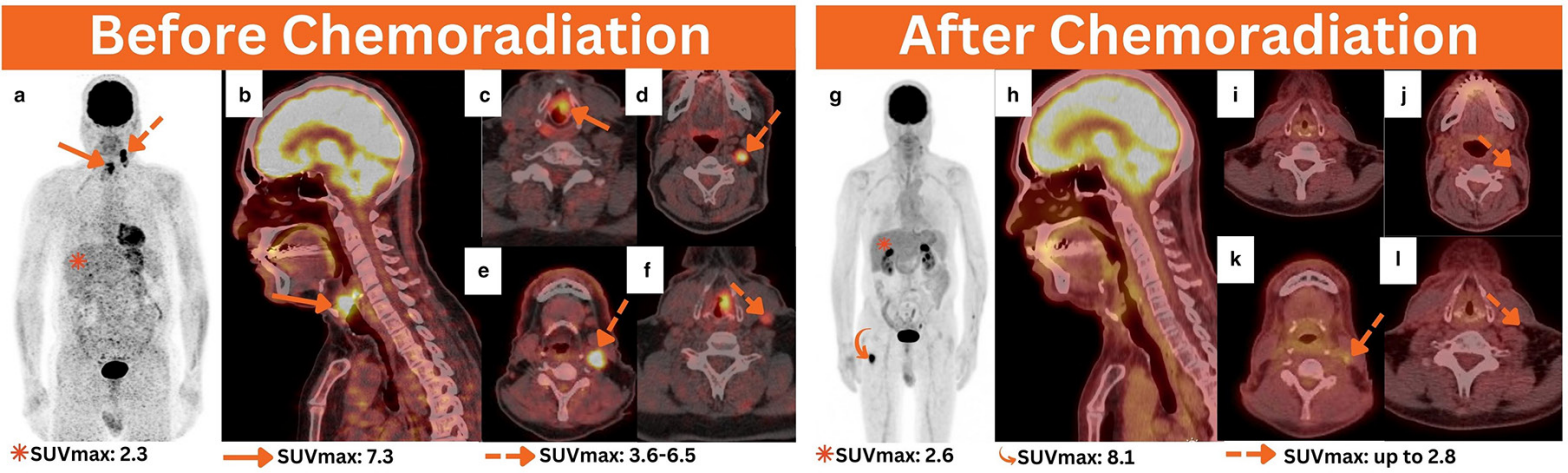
Baseline ^18^F-FDG PET/CT performed before chemoradiation for staging purposes. MIP (a), sagittal (b), and axial ^18^F-FDG PET/CT images (b, c) revealed hypermetabolic left glottic malignancy (arrows). Additionally, axial PET/CT images of the neck (e–f) showed a few ipsilateral hypermetabolic cervical lymphadenopathies involving II–IV levels (dotted arrows). In follow-up, ^18^F-FDG PET/CT performed after chemoradiation for response assessment, MIP (g), sagittal (h), and axial PET/CT images (i–l) revealed evidence of complete resolution in the previous local disease. This was, however, complicated by a distant hypermetabolic intramedullary focus occupying the right proximal femur, as evidenced by the MIP image, necessitating further evaluation (curved arrow). ^18^F-FDG PET/CT, 18-fluorine fludeoxyglucose positron emission tomography/CT; MIP, maximum intensity projection.
